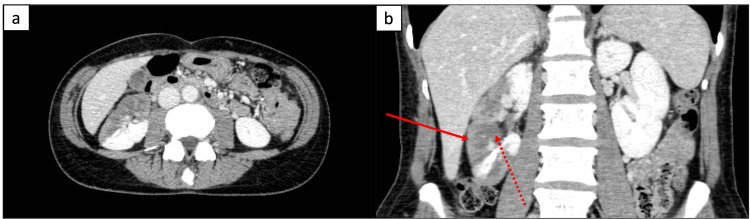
Contrast-enhanced CT of the abdomen showing right renal infarctions; enhancement of the renal cortex was preserved during the late phase. (a) transverse section, (b) coronal section.
